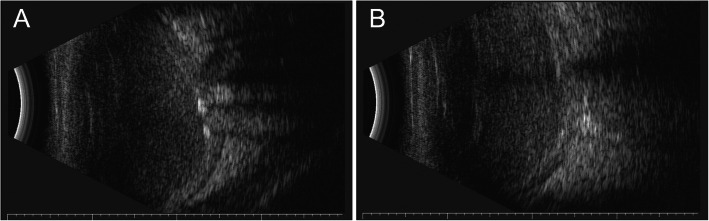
B-scan ultrasound of right eye revealed dense dotted hyperechoic foci in vitreous cavity and retinal detachment
